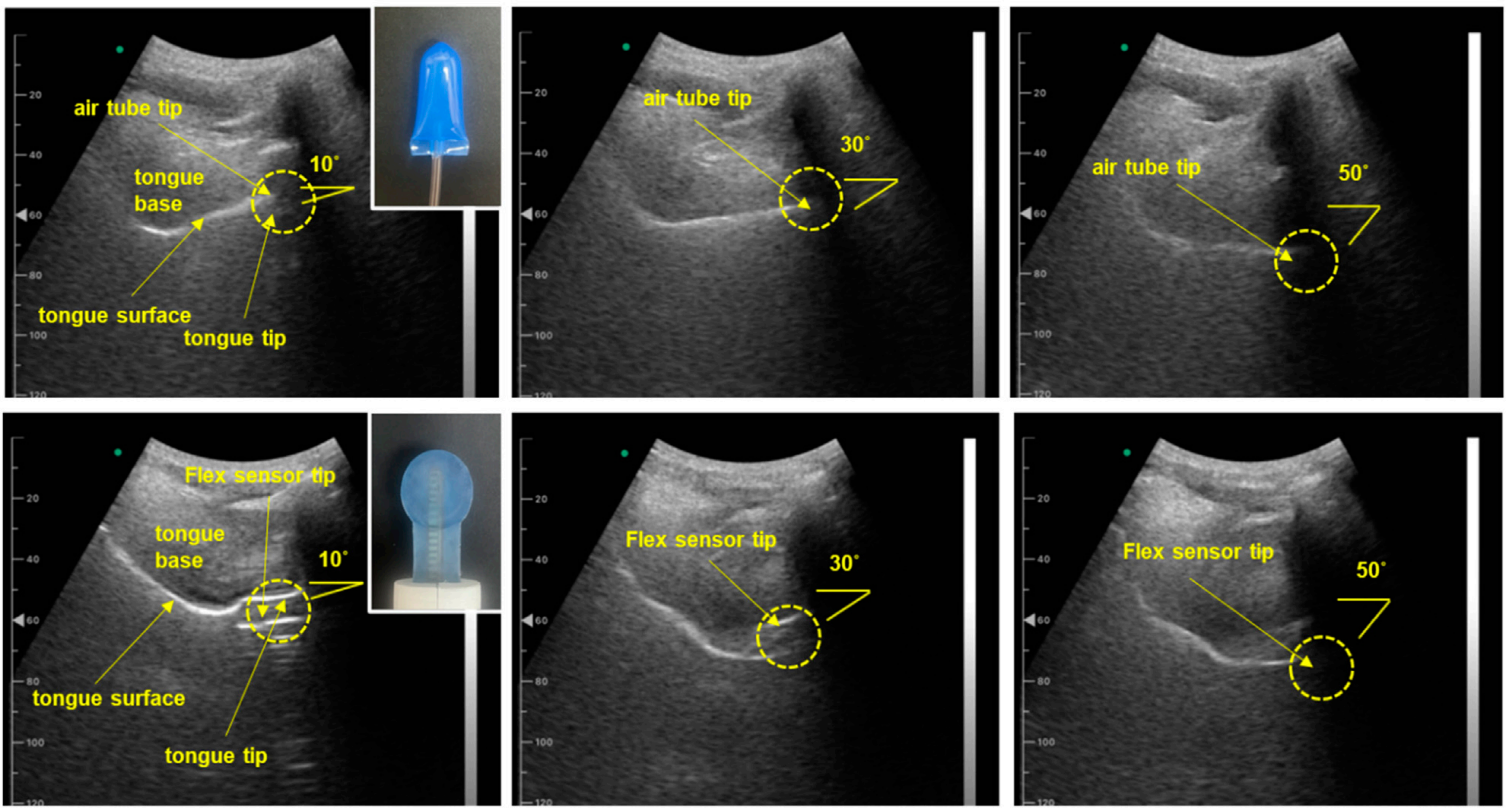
Sonography image for assessing tongue trajectory. (Top row) Subject one wearing the IOPI air flow tube sensor, with images captured at various tongue push-up angles ranging from 10° to 50°. (Bottom row) Subject one wearing the sensor from the proposed oral motor exerciser, with images captured as the participant progressively changed the range of tongue movement. (Participants wore both types of sensors, adjusting their tongue range progressively; angles were confirmed in real-time using sonography sagittal view images.)
